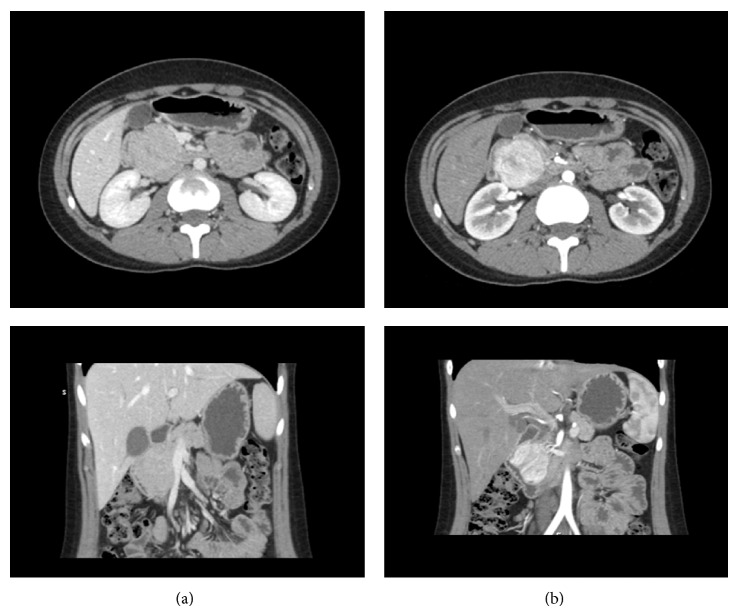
Abdomen computed tomography showing a mass at the head of pancreas. (a) Portal vein phase: isodense appearance of the mass to the rest of the pancreas. (b) Arterial phase: enhancement of the mass.
